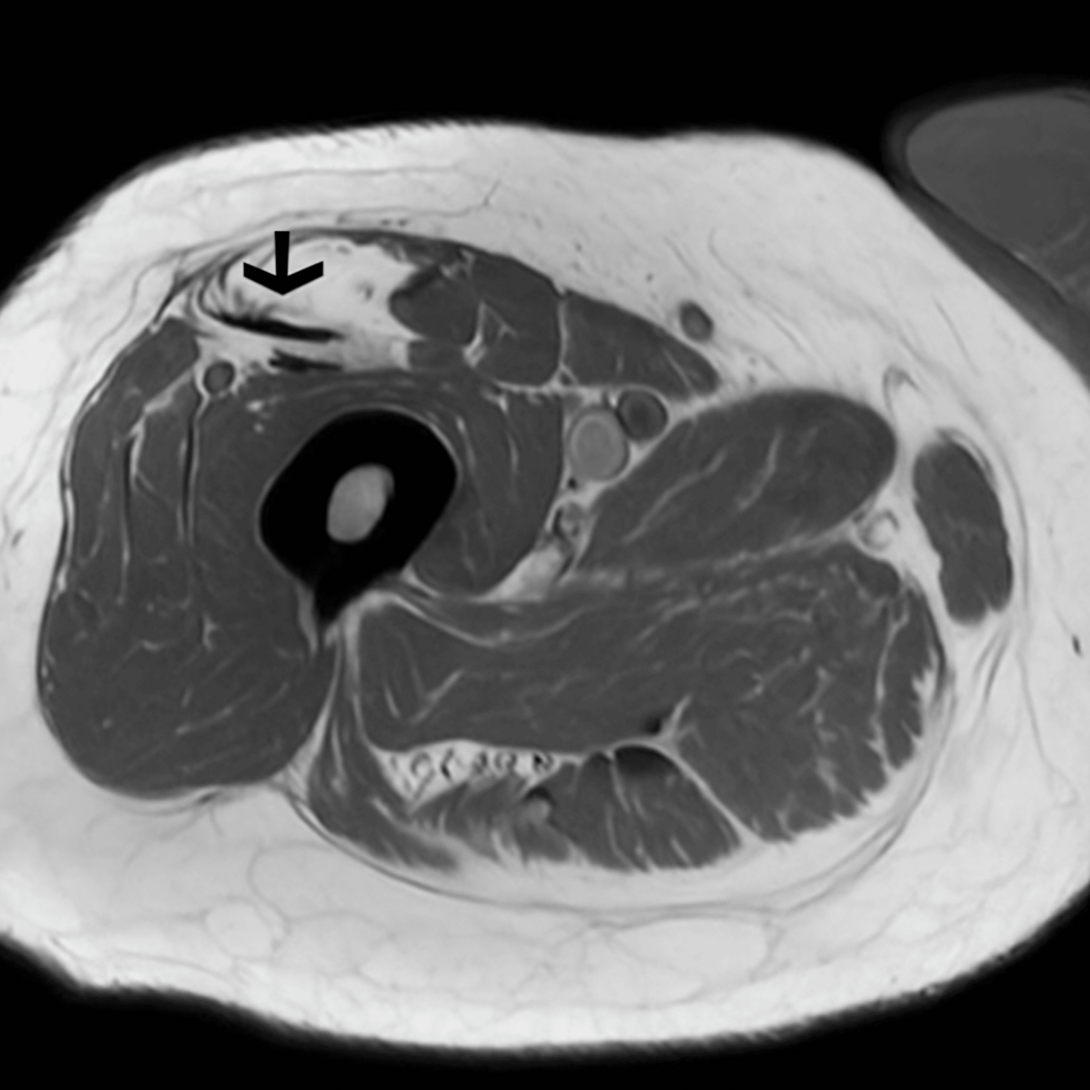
Axial T1-weighted MRI of the right thigh MRI of the right thigh showing fatty infiltration(arrow) of the rectus femoris and muscle atrophy
